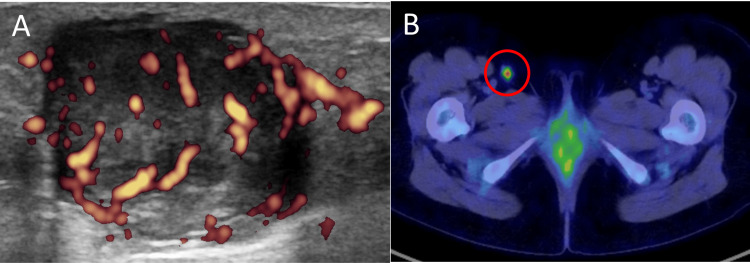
Imaging examination (A) The ultrasound examination revealed a well-circumscribed 3-cm subcutaneous mass with internal blood-flow signal. (B) Postoperative PET/CT suggested a metastasis in the right inguinal lymph node (SUV=4.3), circled in red. PET/CT: positron emission tomography/computed tomography; SUV: standardized uptake value
